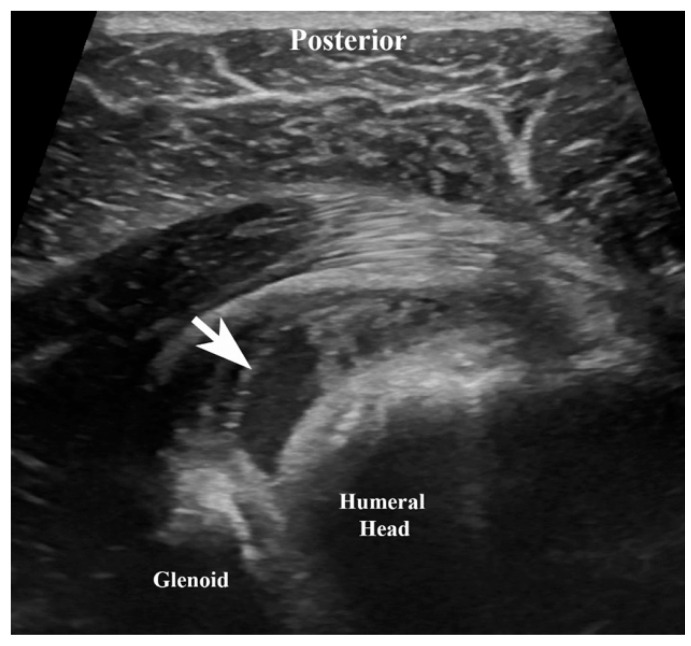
A single ultrasound image depicts the joint effusion targeted for aspiration. This is a view from the posterior shoulder with the arrow pointing to the joint fluid/effusion. The humeral head and glenoid are labeled for anatomic reference.
